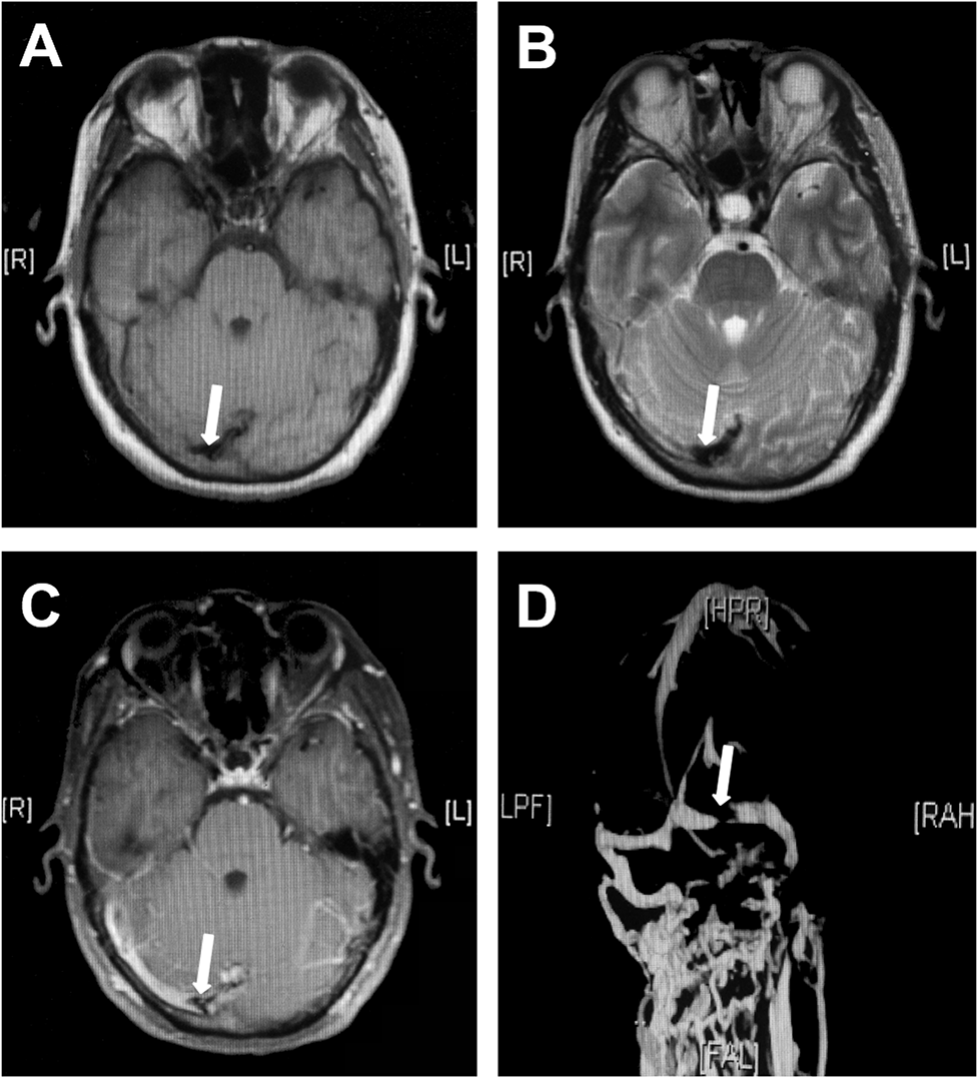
Transverse sinus occlusion without double-track sign. A 38-year old male patient with right transverse sinus occlusion. The thrombus was hypointense on T1WI (A) and T2WI (B), and double-track sign was not observed in right transverse sinus on axial Gd-enhanced T1WI (C). Transverse sinus occlusion was confirmed on MRV which showed a lack of blood flow signal in the right transverse sinus (D) (Time from MRI to MRV was 2 hours).
